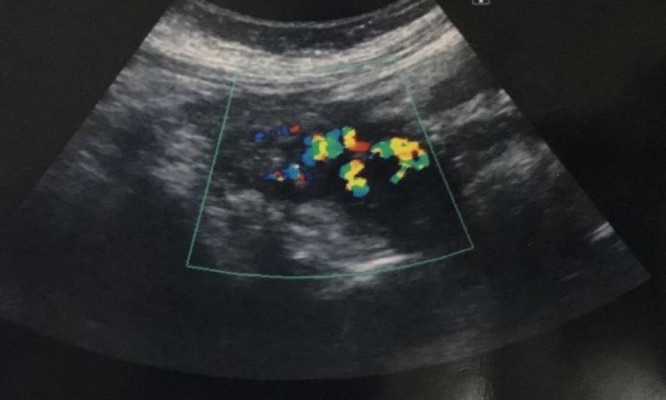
Color flow Doppler evaluation of the uterus demonstrating increased blood flow at left uterine artery region (blue box).
